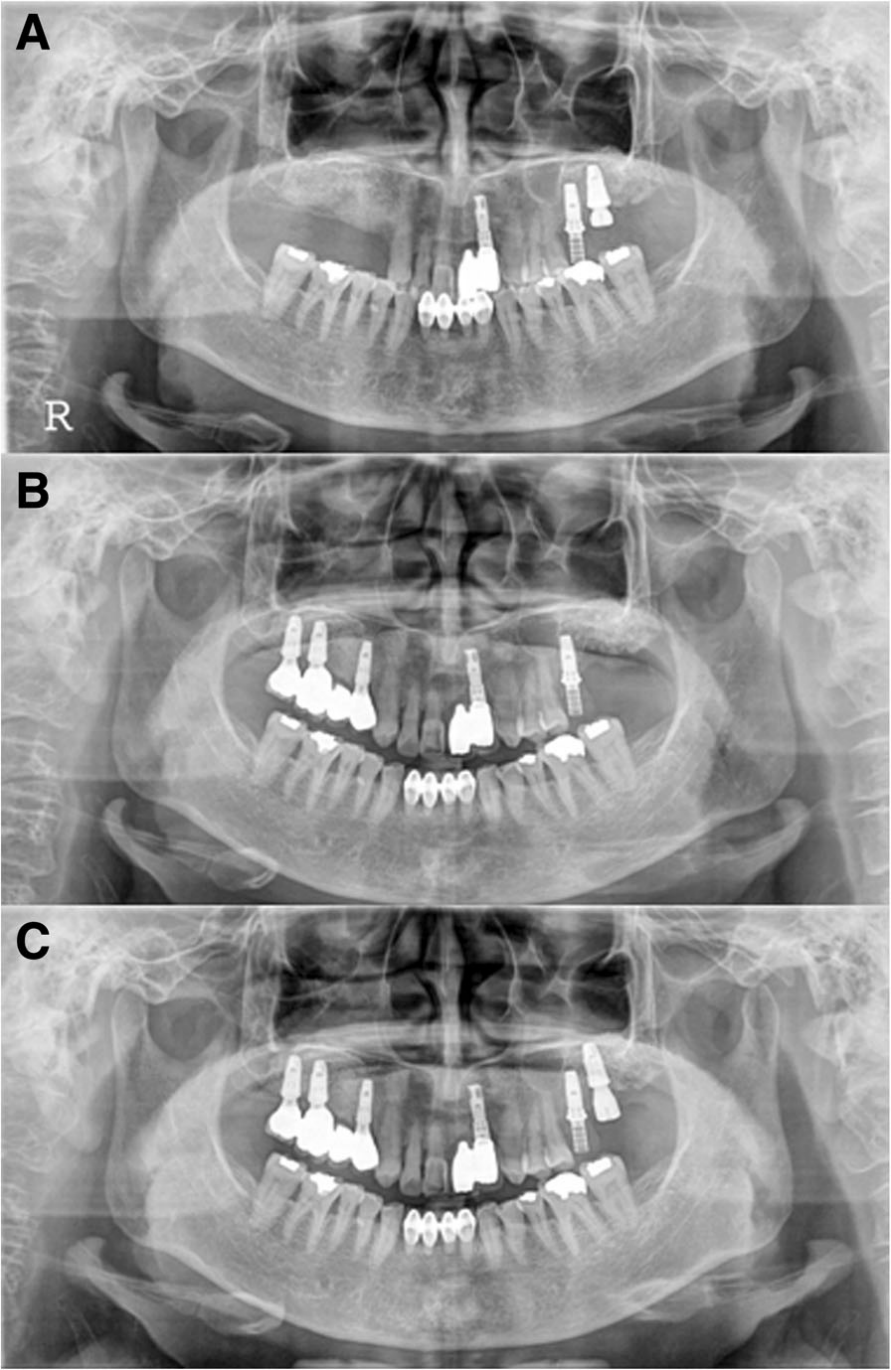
F2, panoramic radiograph, a #27i second installation, b #27i second failure, preoperative panoramic radiograph, c #27i, third installation
